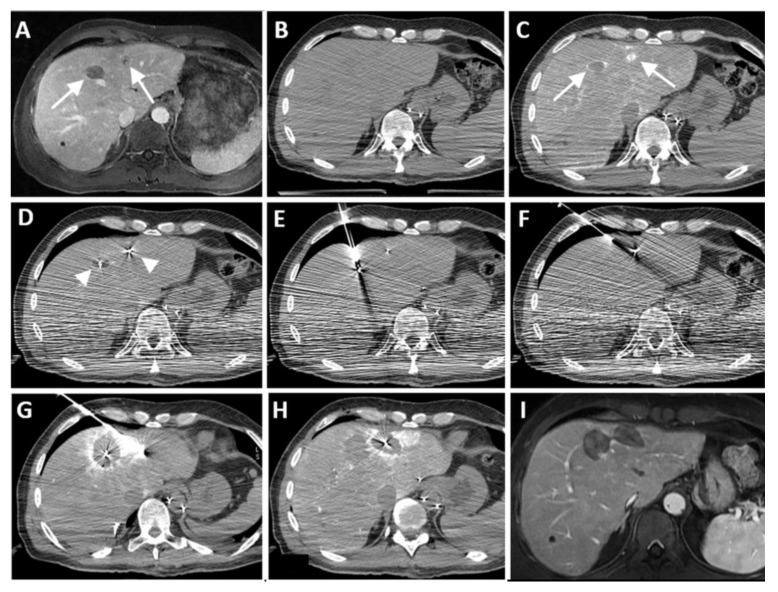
Identification of colorectal liver metastases. Two colorectal liver metastases (arrows) visible on diagnostic contrast-enhanced MRIs (A). During the procedure, the lesions were not seen on non-enhanced CT (B). After catheterization of the common hepatic artery, the lesions (arrows) became clearly visible as enhancing rings after the injection of 4 mL Xenetix 300^®^ contrast (Iobitridol 300 mg Iodine/mL, Guerbet, Villepinte, France) (C). Additionally, in this case, 0.018” coils (arrow heads) (Tornado, Cook Medical) have been inserted via a 22 G Chiba needle (Cook Medical, Bloomington, IN, USA) in the middle of the lesions as a fiducial marker and to facilitate post-ablation safety margin assessment (D) [22]. A carboxypneumothorax was being created prior to the actual RFA procedure (E,F) (Cool-tip™, Medtronic-Covidien, Boulder, CO, USA). Another 4 mL contrast was injected after each ablation to assess the created ablation zone (G,H). A follow-up MRI after two months showed no signs of local tumor progression (I).
